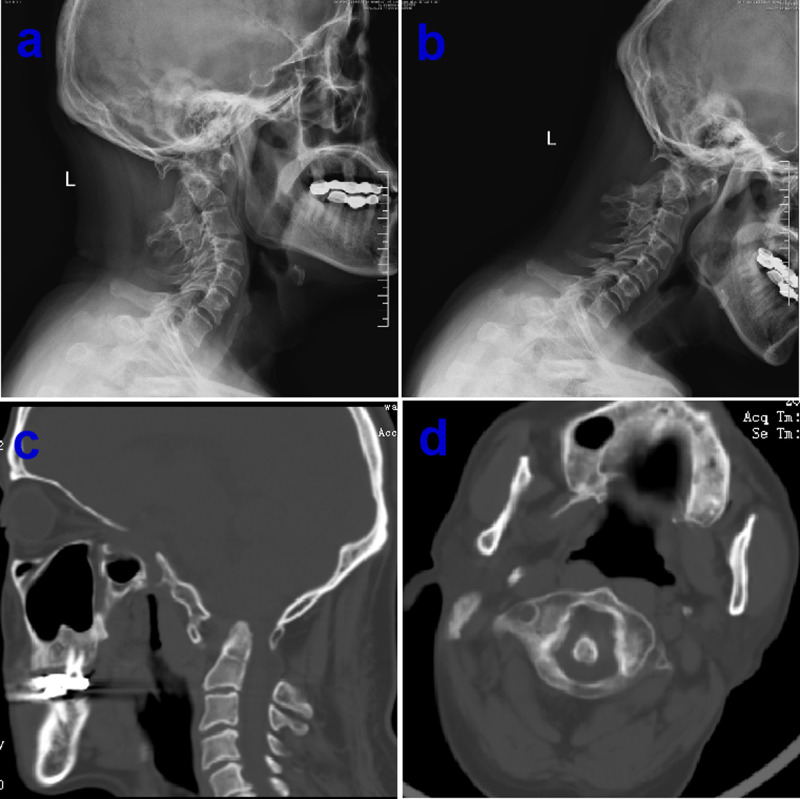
Preoperative over-extension/flexion dynamic X-ray showing normal bone structure of atlantoaxial dislocation, basilar invagination and assimilation of atlas (A and B), computerized tomography (CT) examination confirm concomitant multiple bony abnormalities in the same patient (C and D).
